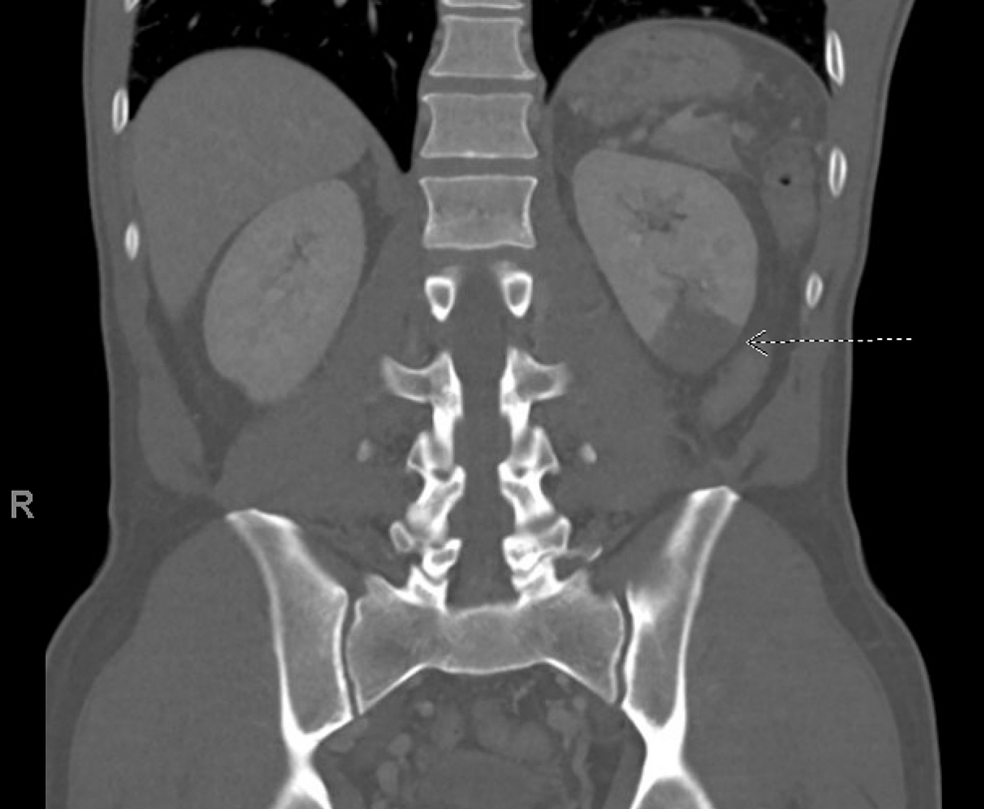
CT of the abdomen with the white arrow pointing at the left inferior pole hypodensity concerning for infarction
